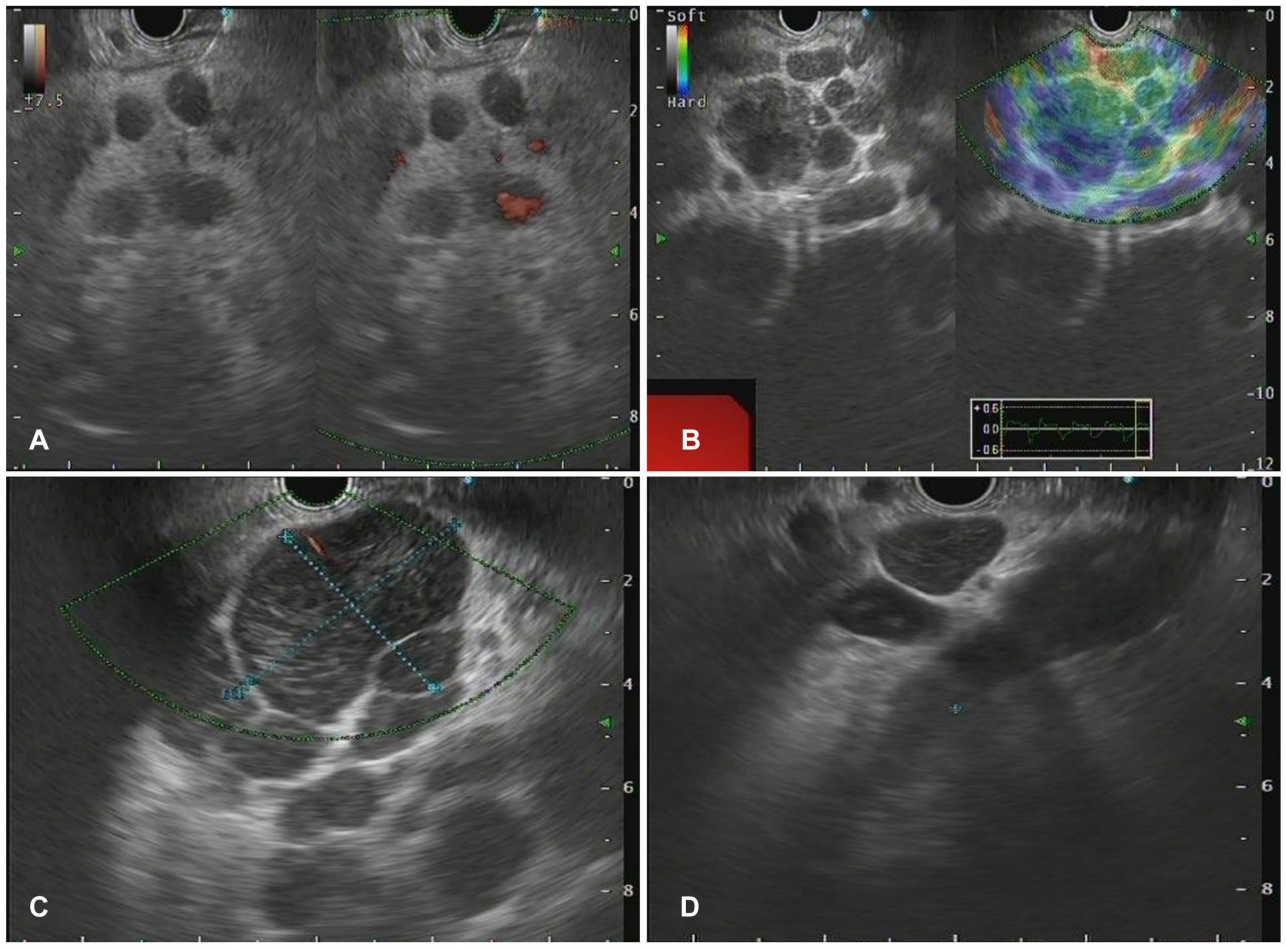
The Endoscopic ultrasonography image features for lymphoma. (A) A case of NKT cell lymphoma. EUS showed circular hypoechoic nodules with partial fusion, and no obvious blood flow signal was observed by Doppler. (B) In the case of follicular lymphoma, EUS showed multiple hypoechoic nodules and partial fusion. (C) One case of diffuse large B-cell lymphoma. EUS showed multiple hypoechoic nodules, Doppler showed a few blood flow signals, and elastic imaging suggested a hard texture. (D) A case of vascular immunoblastic T-cell lymphoma. EUS showed multiple circular hypoechoic nodules with partial fusion.
